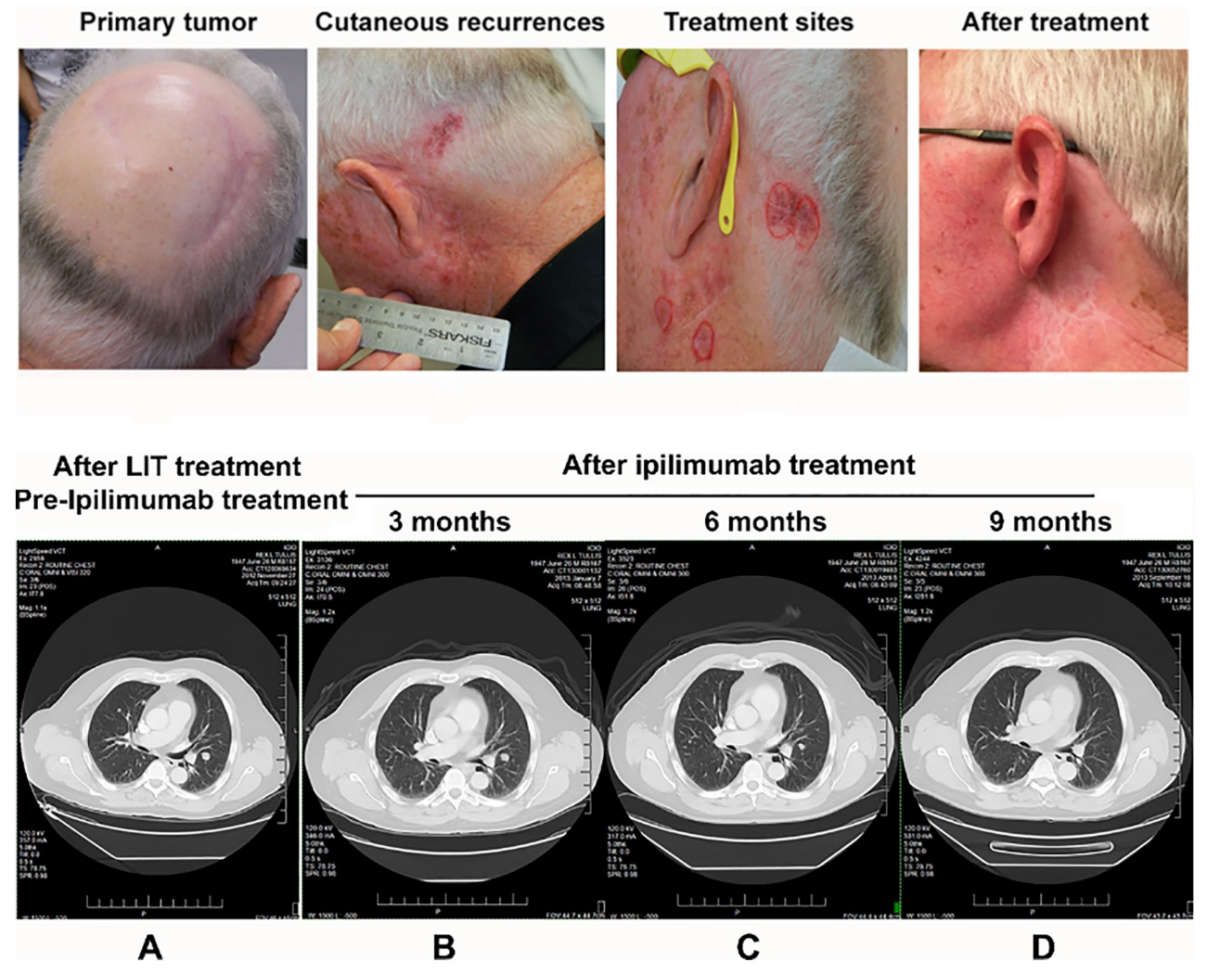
Photographs and CT images of a patient with stage IV melanoma during treatment. Upper: Photographs of the LIT treatment areas around the left ear. A. The primary tumor site with only surgical scars visible. B. The areas of biopsy-proven cutaneous recurrences around 2 surgical scars from the biopsy sites. Scalp hair has been trimmed away from the scalp recurrence site to make superficial laser therapy more effective. Reddish areas around the surgical scars represent melanoma deposits in the superficial dermis. C. Areas circled in red represent the 4 treatment sites selected for LIT. All other areas of regional involvement completely resolved during the 4-week treatment phase, which is typically seen with superficial LIT. D. The treatment area after LIT. Three months later, the LIT treatment completely cleared all cutaneous melanoma around the left ear. Bottom: CT images of the patient taken 3 months apart showing the same level in the thorax. A. The image was taken before ipilimumab treatment (2 months following LIT) and demonstrates the size and location of the pulmonary metastases. B-C. The images show shrinkage of the pulmonary metastases 3 months (B) and 6 months (C) after completion of the combination of LIT and ipilimumab. D. The image shows that pulmonary metastases were completely resolved 9 months after completion of the combination of LIT and ipilimumab. The figure is adapted with permission from 35, copyright 2017 Wiley‐VCH Verlag GmbH & Co. KGaA, Weinheim.
